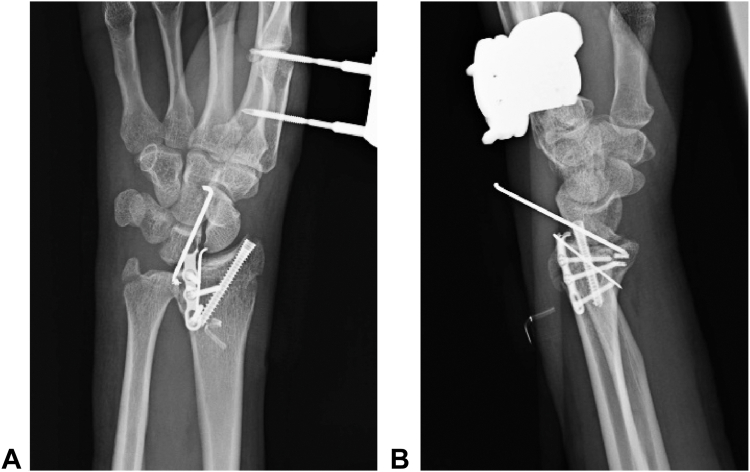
The graft is fixed with a plate and Kirschner wire. A Post-transplant anteroposterior radiograph. B Post-transplant lateral radiograph.
